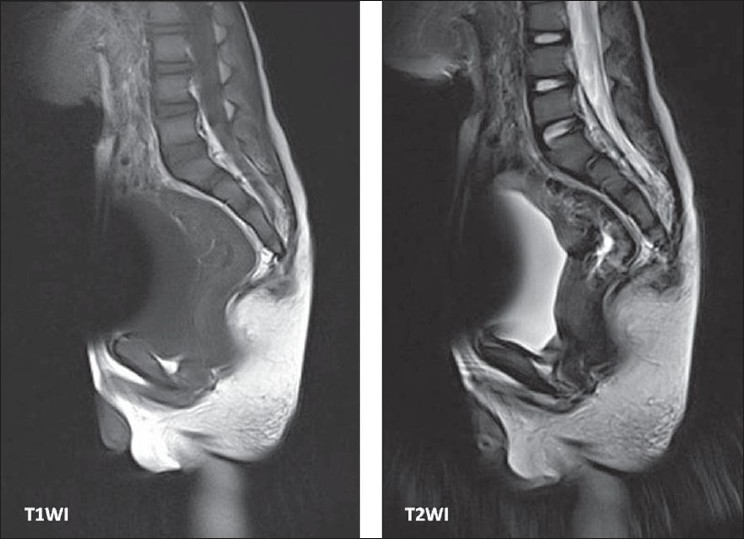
Postoperative sagittal T1-weighted and T2-weighted images
